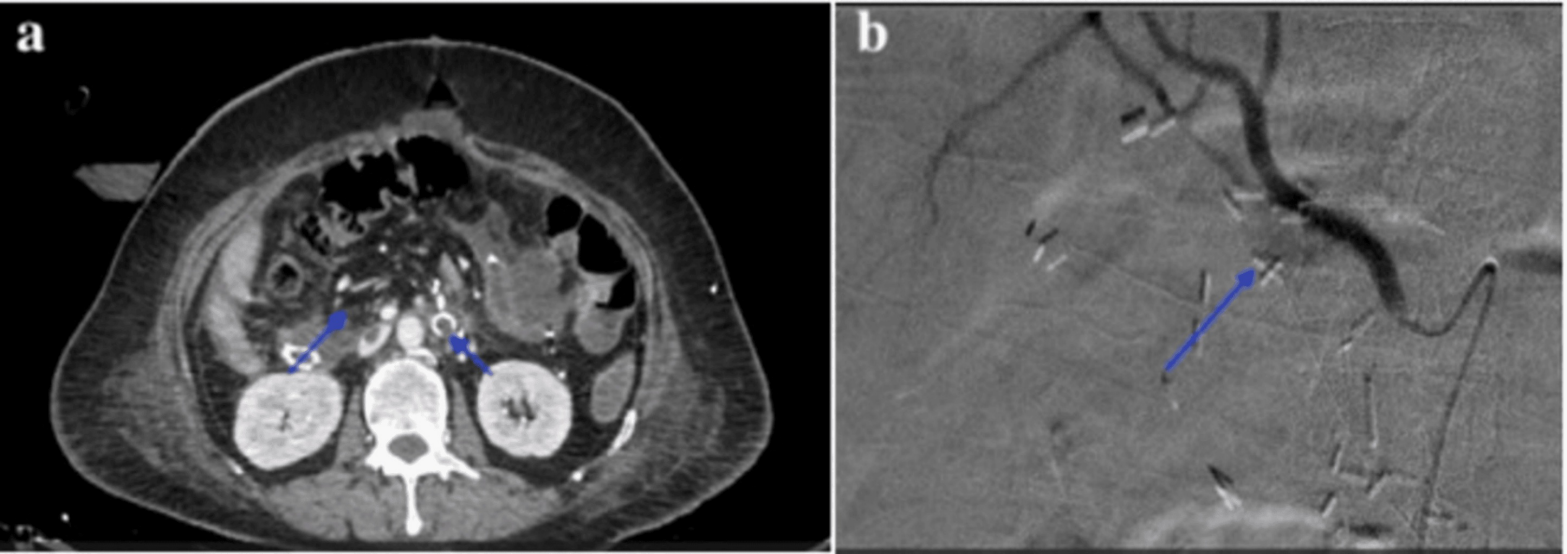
(a) Axial contrast-enhanced CT image showing a pseudoaneurysm (yellow arrow) near the gastroduodenal artery stump with contrast extravasation. (b) Digital subtraction angiography (DSA) image confirming active contrast extravasation from the GDA stump pseudoaneurysm.
